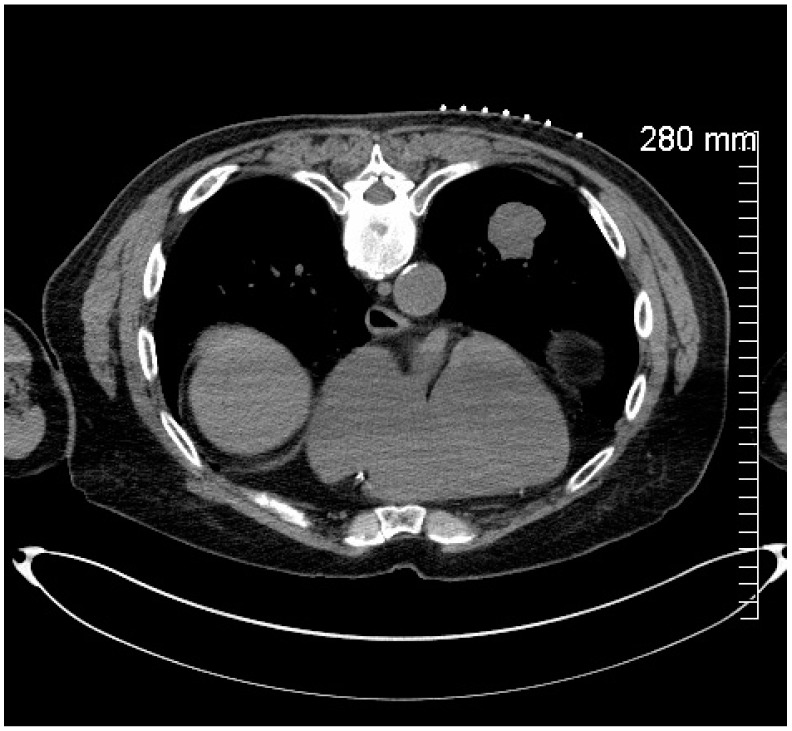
Pre-procedure CT chest without contrast demonstrating a solitary 3.2 × 3.1 cm left lower lobe lung mass in an 82-year-old male with a history of scalp melanoma pre-senting with solitary 3.2 × 3.1 cm left lower lobe lung mass.
